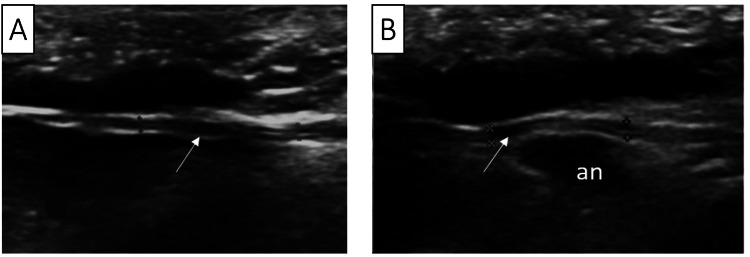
Ultrasound imaging of the deep branch of the ulnar nerve on the unaffected (A) and affected (B) hands. In image A, the nerve path is straight, with no additional formations in the surrounding soft tissues. In image B, the nerve path is irregular, displaced by a round anechoic formation, a pseudoaneurysm.
